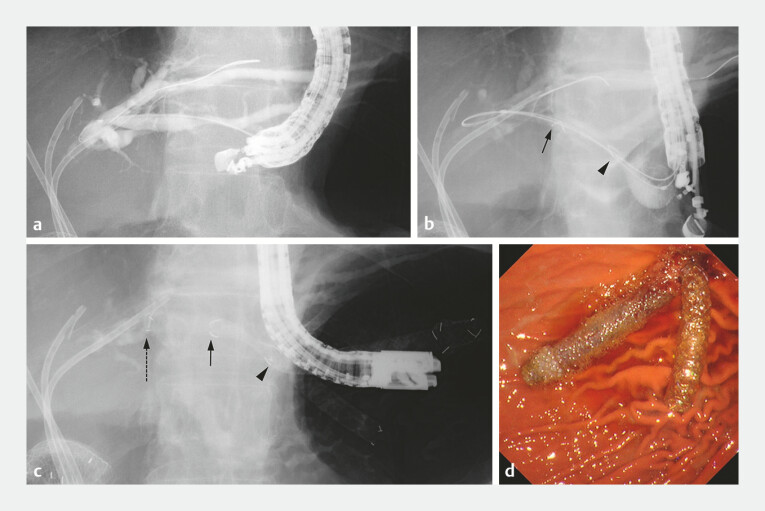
Fluoroscopic and endoscopic images during endoscopic ultrasound-guided hepaticogastrostomy.
